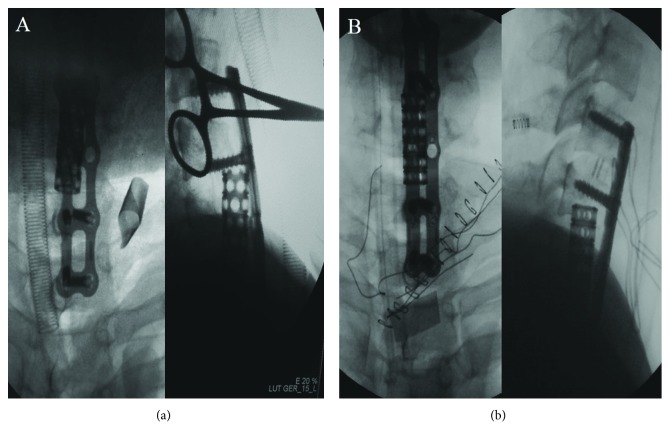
(a) X-ray during the first surgery. (b) X-ray during the second surgery.
